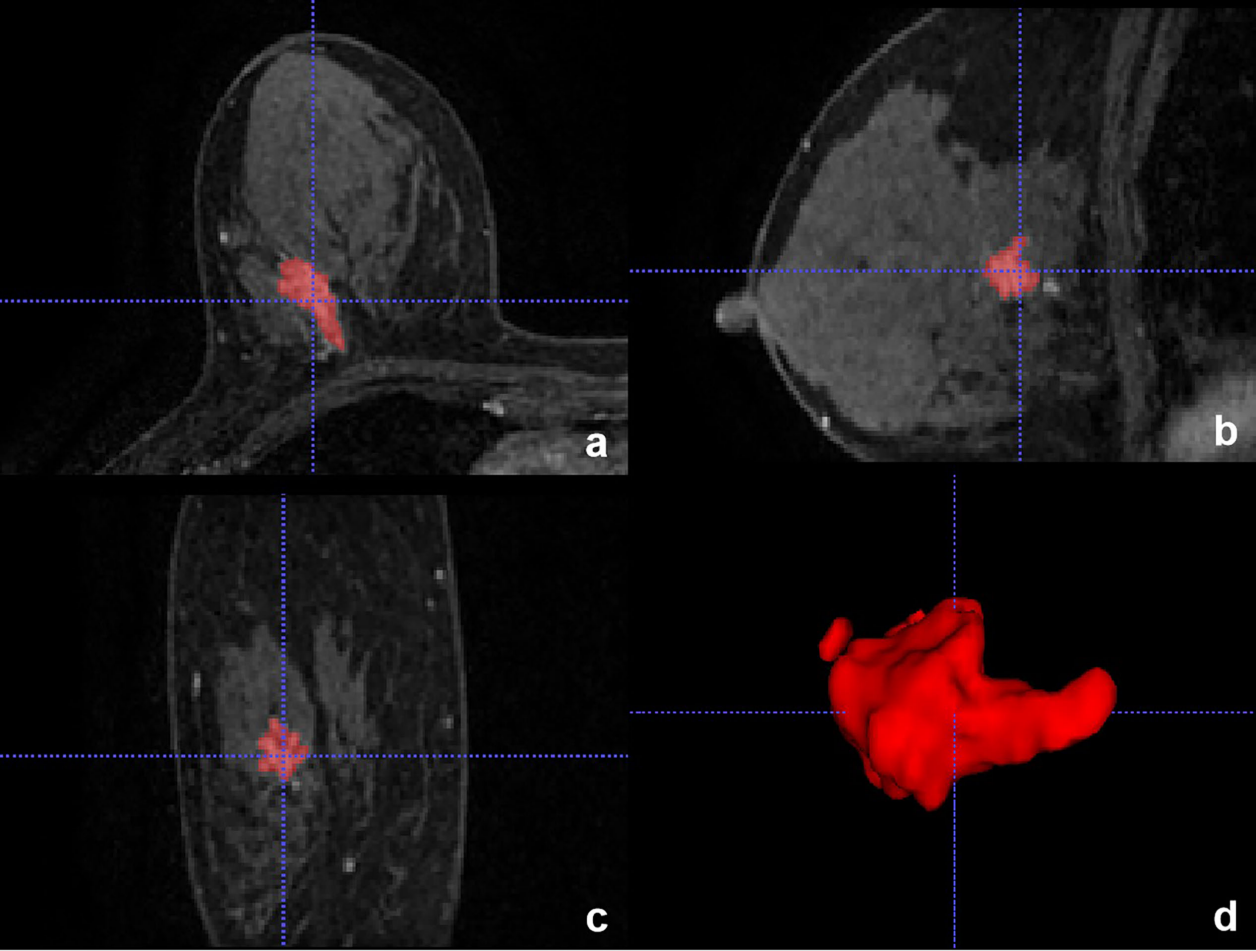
Invasive breast cancer in a 57-year-old woman in the testing set with true-positive result. (A-C) Axial, Sagittal, and Coronal T1-weighted postcontract image showed non-mass enhancement in the right breast. (D) 3D reconstruction of the lesion.
